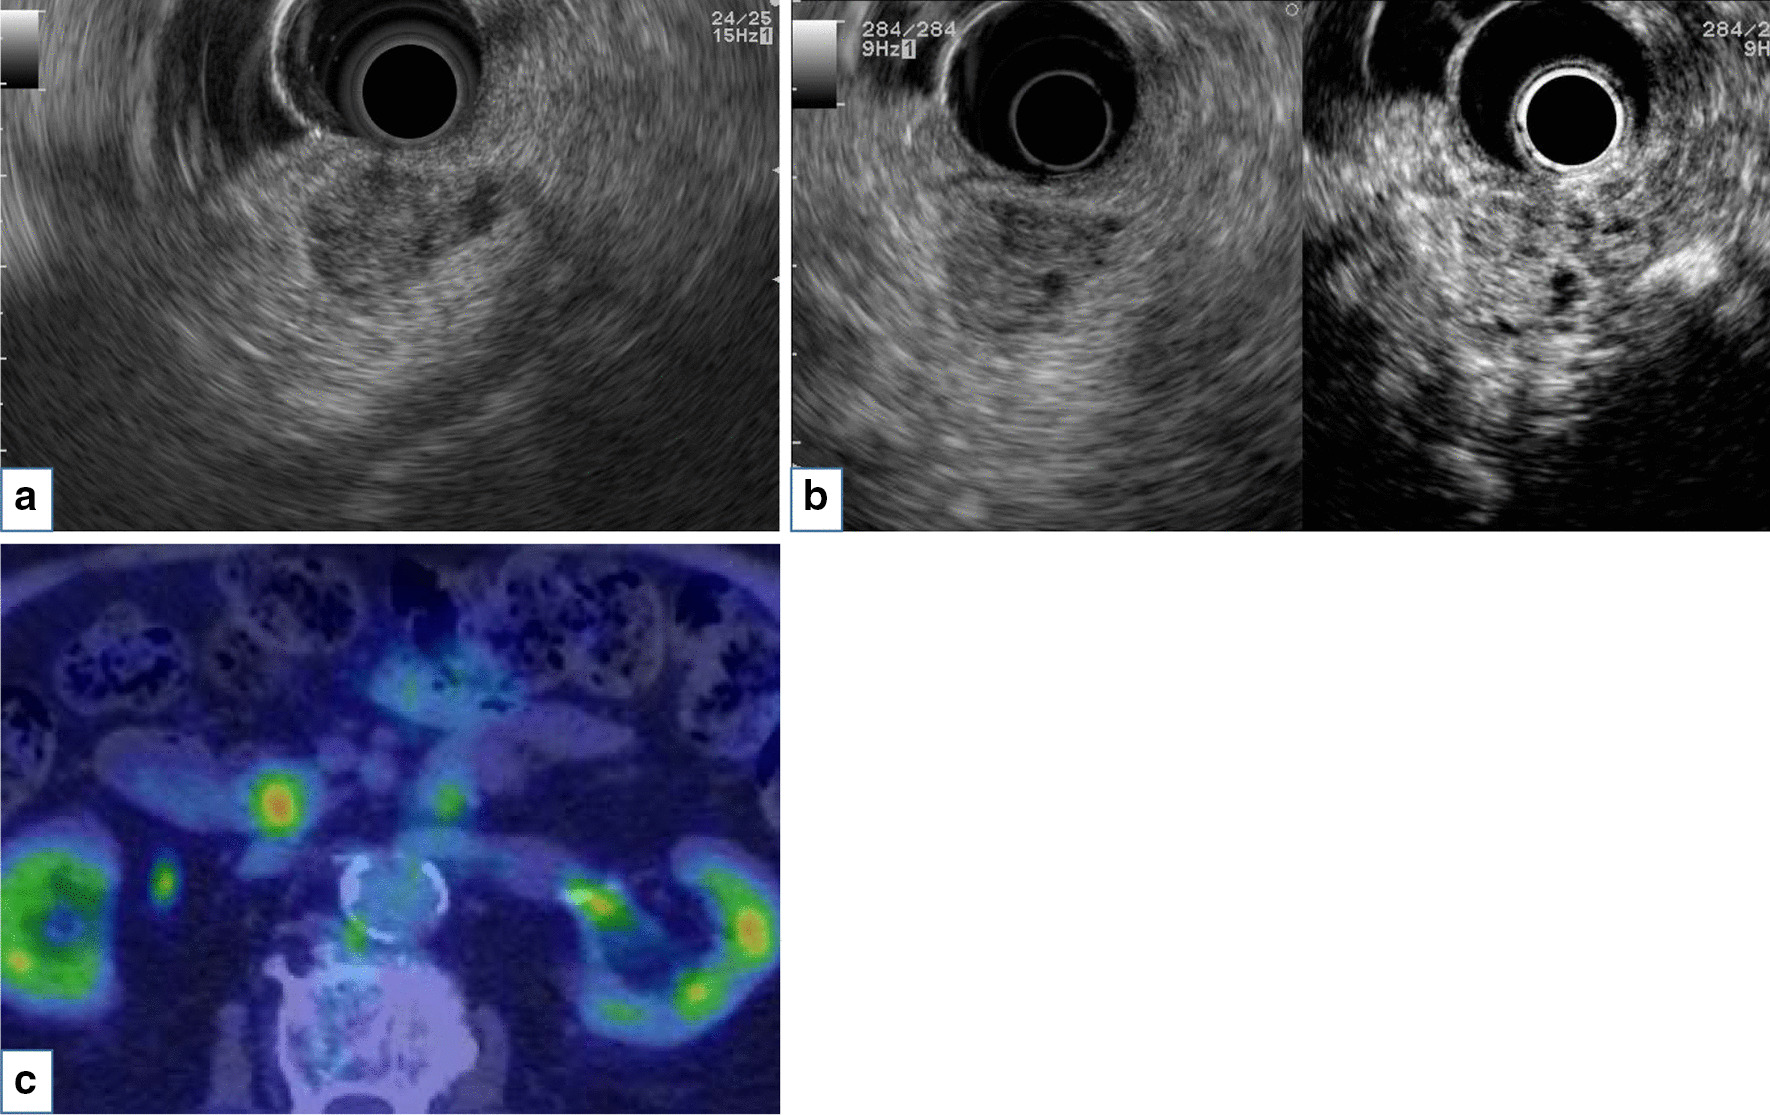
Endoscopic ultrasonography showed 15 mm round hypo echoic mass with fluid collection in its margin (a) and contrast enhanced-ultrasonography using Sonazoid showed iso or high vascularity (b). Positron emission tomography revealed high accumulation of 2-[^18^F] fluoro-2-deoxy-D-glucose as 4.53 of max standardized uptake value (c)
